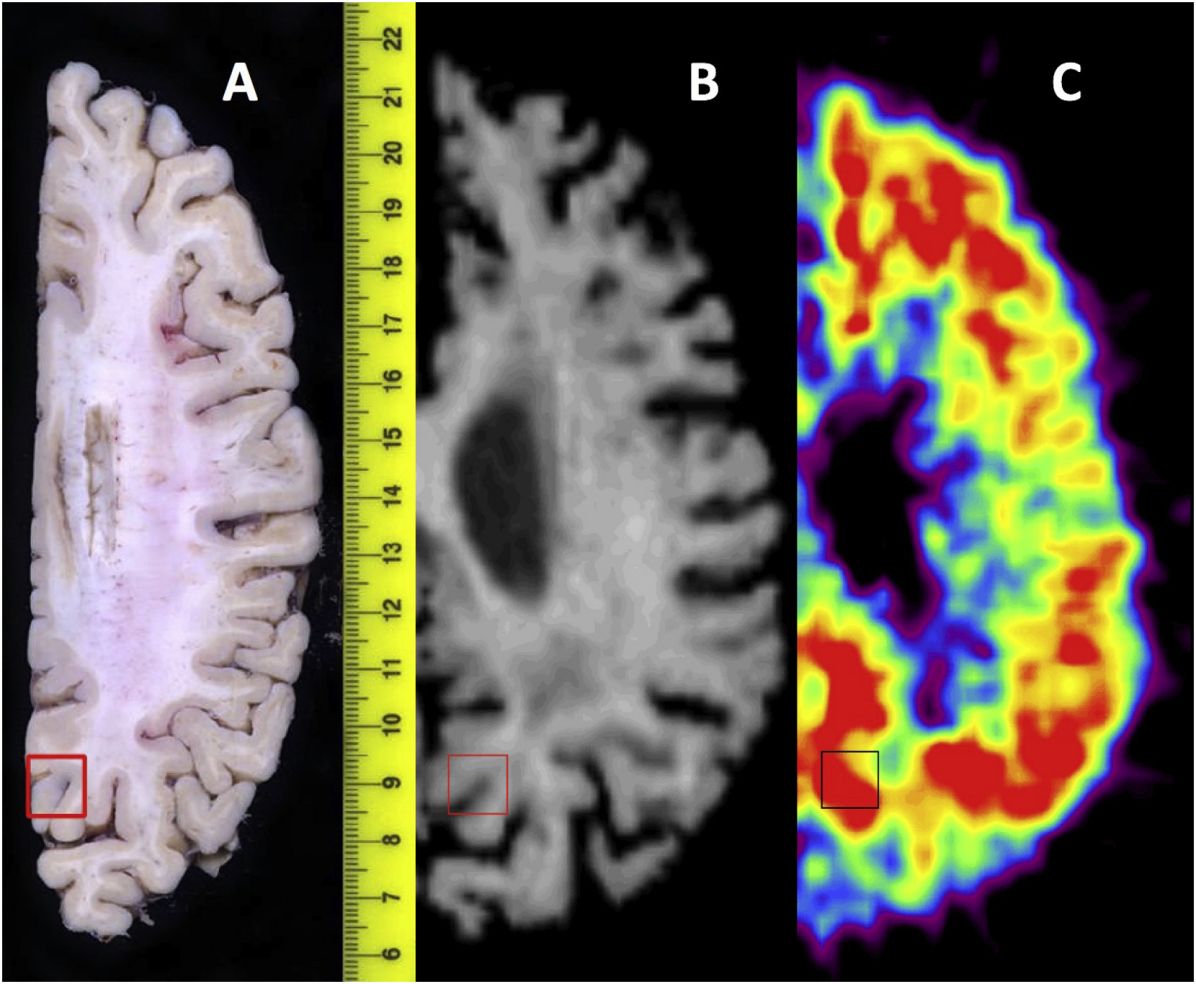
Snapshot of precuneus VOI matching between post-mortem axial tissue block and matching in vivo MR image for Case#07. From left-to-right, (A) is the post-mortem autopsy tissue block with excised region boxed, (B) is the in vivo MR image with VOI hand-drawn to match that in the autopsy photo, and (C) is the [C-11]PiB SUVR image with the hand-drawn VOI overlaid.
